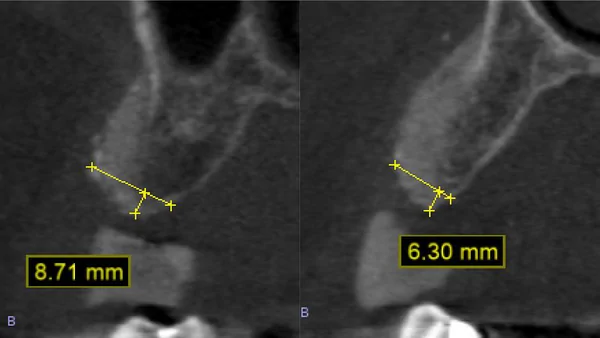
CBCT image 6 months after bone augmentation, 6.30 mm in width was observed on site #12 and 8.71 mm on site #14.
